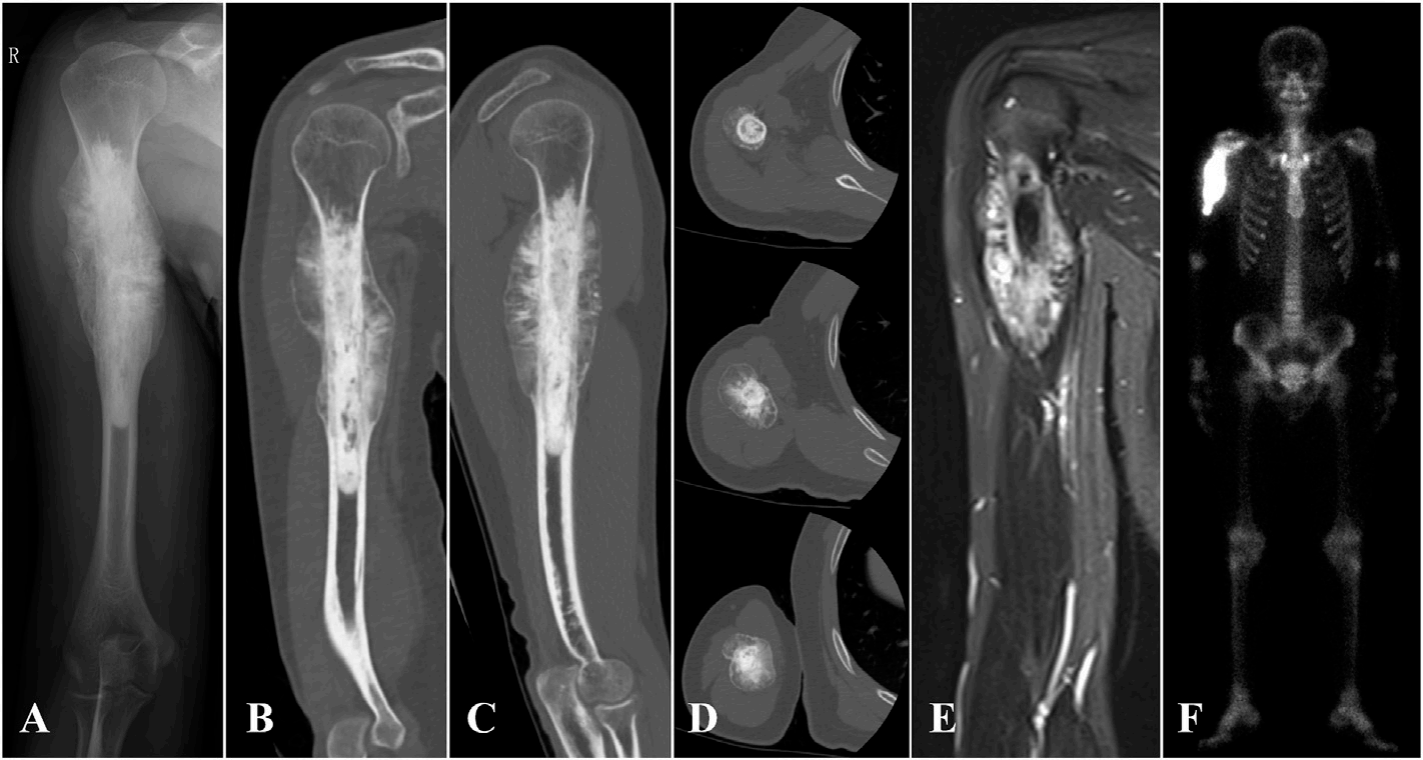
(A) X-ray, (B–D) 3D CT, (E) MRI, and (F) SPE/CT of case 1 with proximal humerus osteosarcoma are shown.
